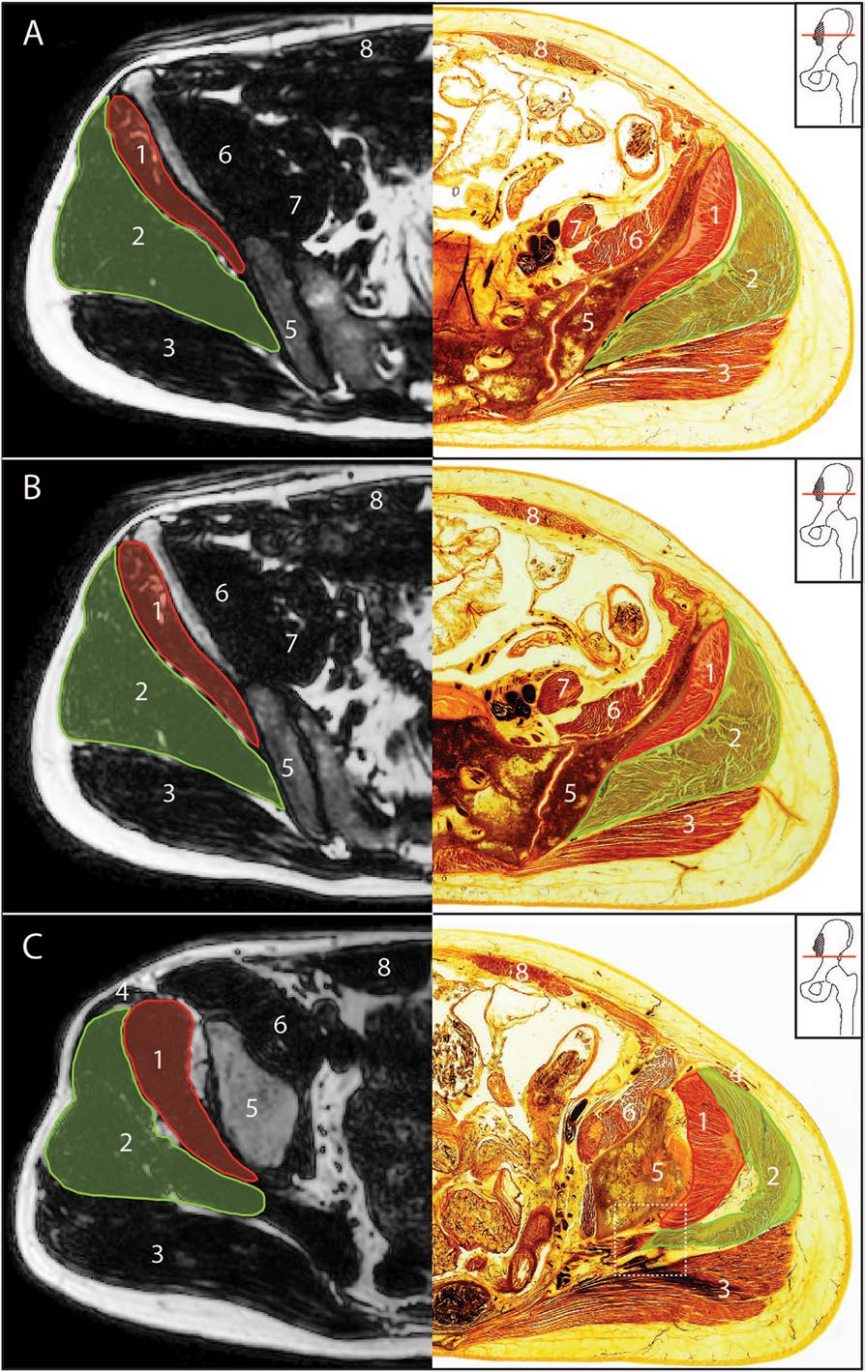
Axial DIXON sequence MRI and E-12 anatomical plastinate comparison at anatomical levels for cross sectional area measurement above the hip joint. A At the level of anterior superior iliac spine B Halfway between the iliac crest and the superior tip of the greater trochanter C Anterior inferior iliac spine; square dotted box surrounds enlarged morphological region of interest (Fig. 4); 1- gluteus minimus; 2- gluteus medius; 3- gluteus maximus; 4- TFL; 5- ilium; 6- iliacus; 7- psoas major; 8- rectus abdominis
